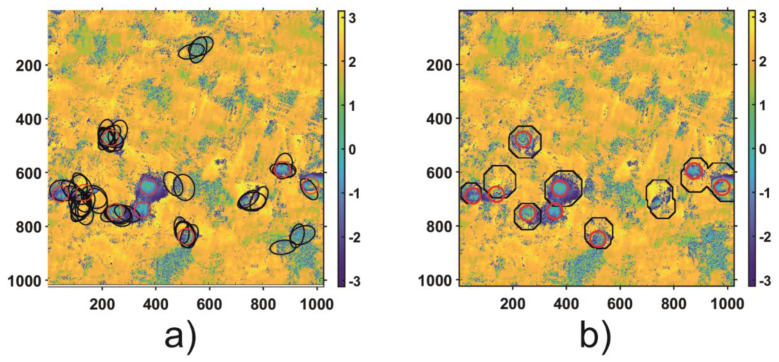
Results of subsidence trough detection using HT (black elliptical shapes on (a)) and CT coefficient analysis (black contours on (b)) computed for Area 1. The true location of subsidence troughs obtained from visual detection is depicted with red circles on both subpictures.
